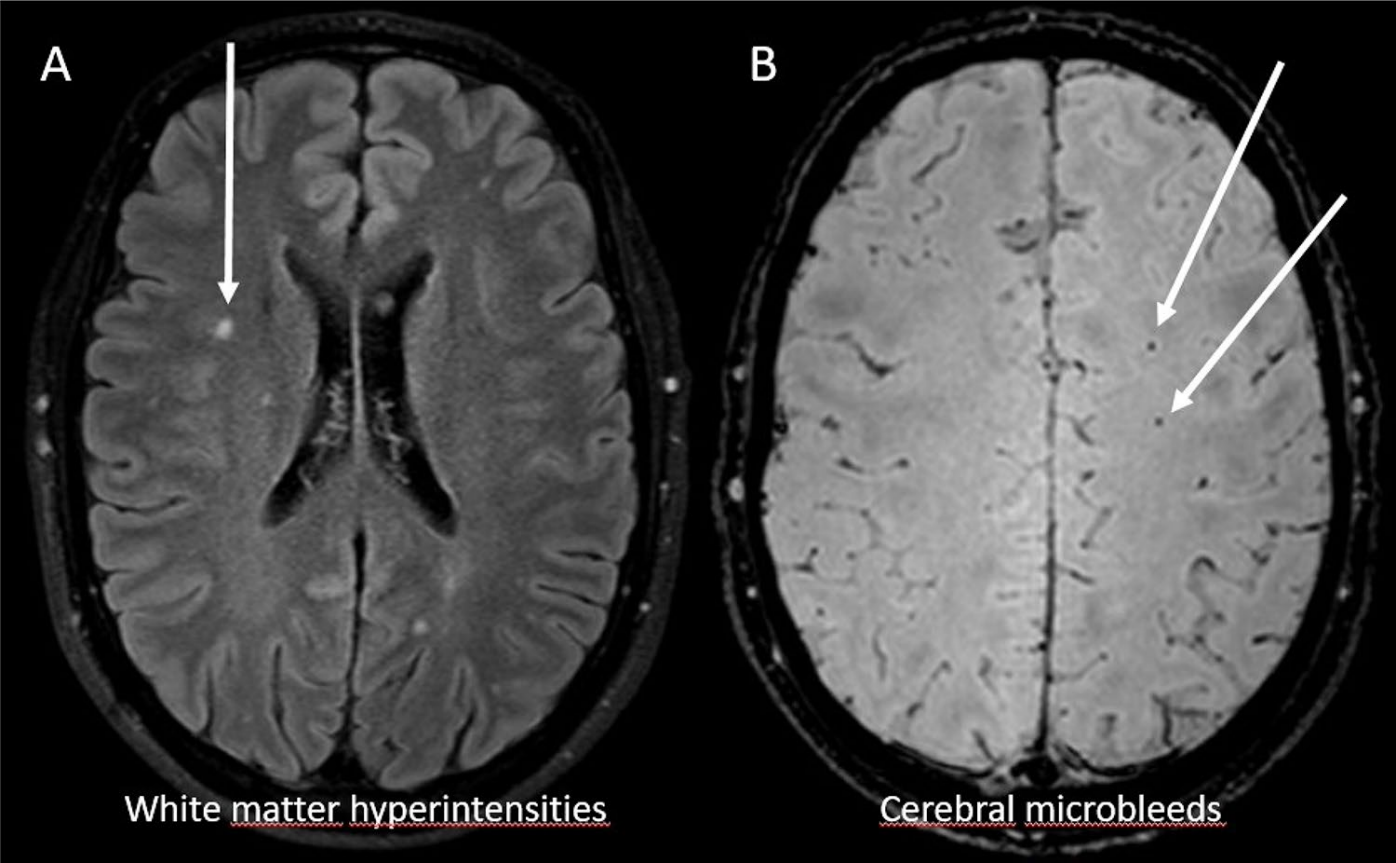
MRI findings of cerebral small vessel disease. Fluid attenuated inversion recovery image (FLAIR) with white matter hyperintensity (arrow) (a). Susceptibility weighted image (SWI) with cerebral microbleeds (arrows) (b)
